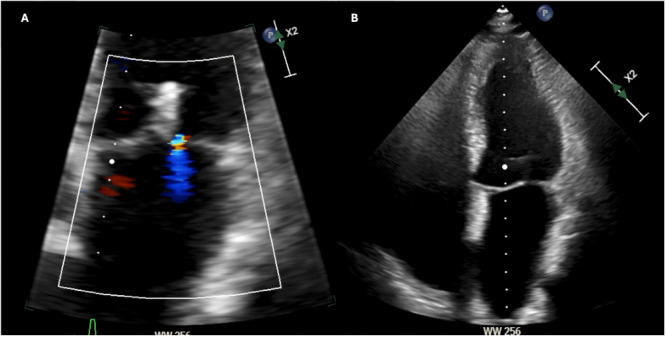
Transthoracic echocardiography at 9‐year follow‐up. Transthoracic imaging at 9‐year follow‐up showed mild residual mitral regurgitation (A) and left ventricular reverse remodeling (B). [Color figure can be viewed at wileyonlinelibrary.com]
